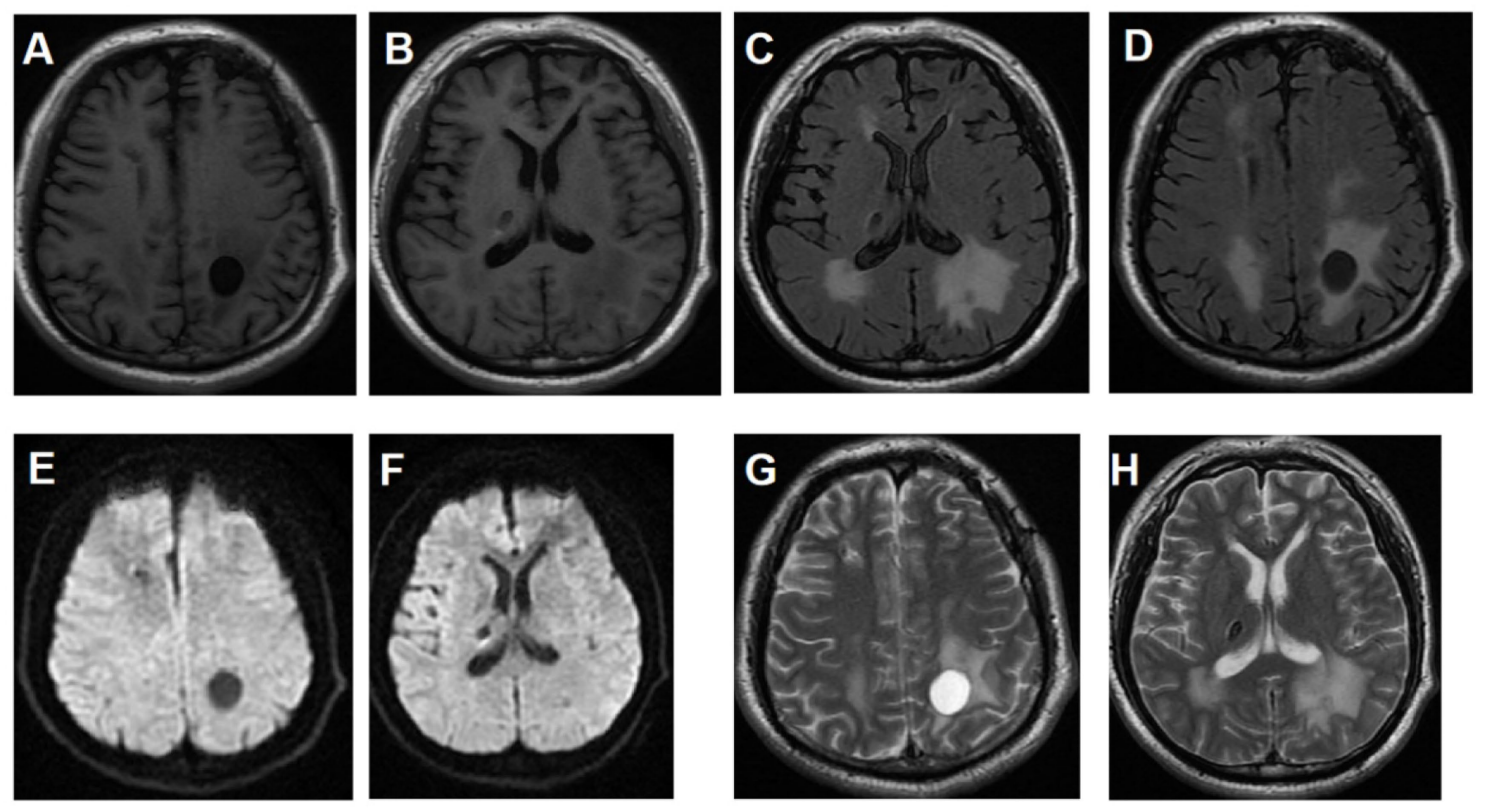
Cranial MRI review of patient 2019 April two years after surgery. (A,B) MRI T1 (C,D) MRI T2 (E,F) MRI diffusion weighted imaging (G,H) MRI AXIFSET2WI2. Bilateral frontal lobe cysts disappeared, the volume of left occipital lobe cyst increased compared with before, and the calcification of right thalamus remained unchanged.
